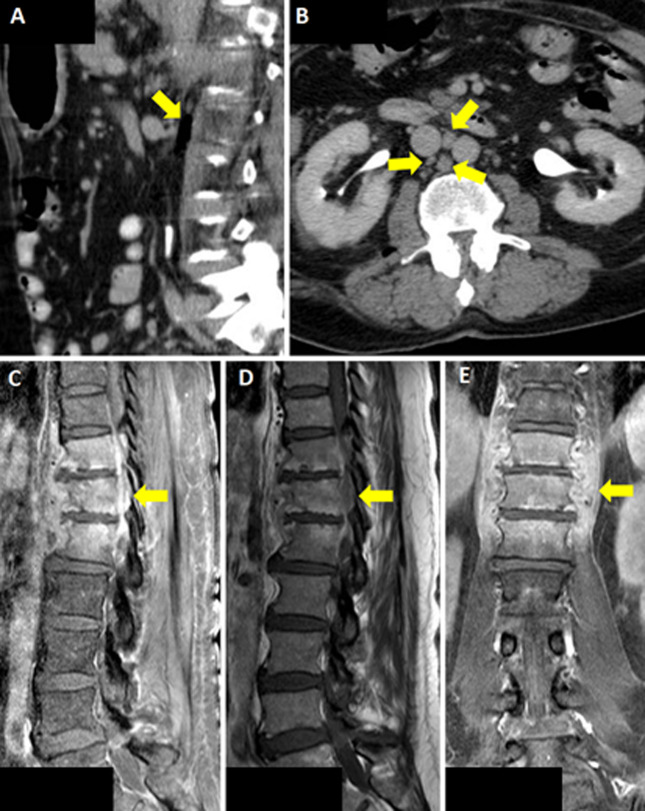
CT scan of the abdomen showing arrows pointing to: A) air in the retroperitoneal space; B) interaortocaval adenopathies; C,D) sagittal lumbar spine MRI, arrows pointing to T11-L1 with spondylodiscitis changes and associated paraspinal collection; E) coronal lumbar spine MRI, arrow pointing to T11-L1 with spondylodiscitis changes and associated paraspinal collection
